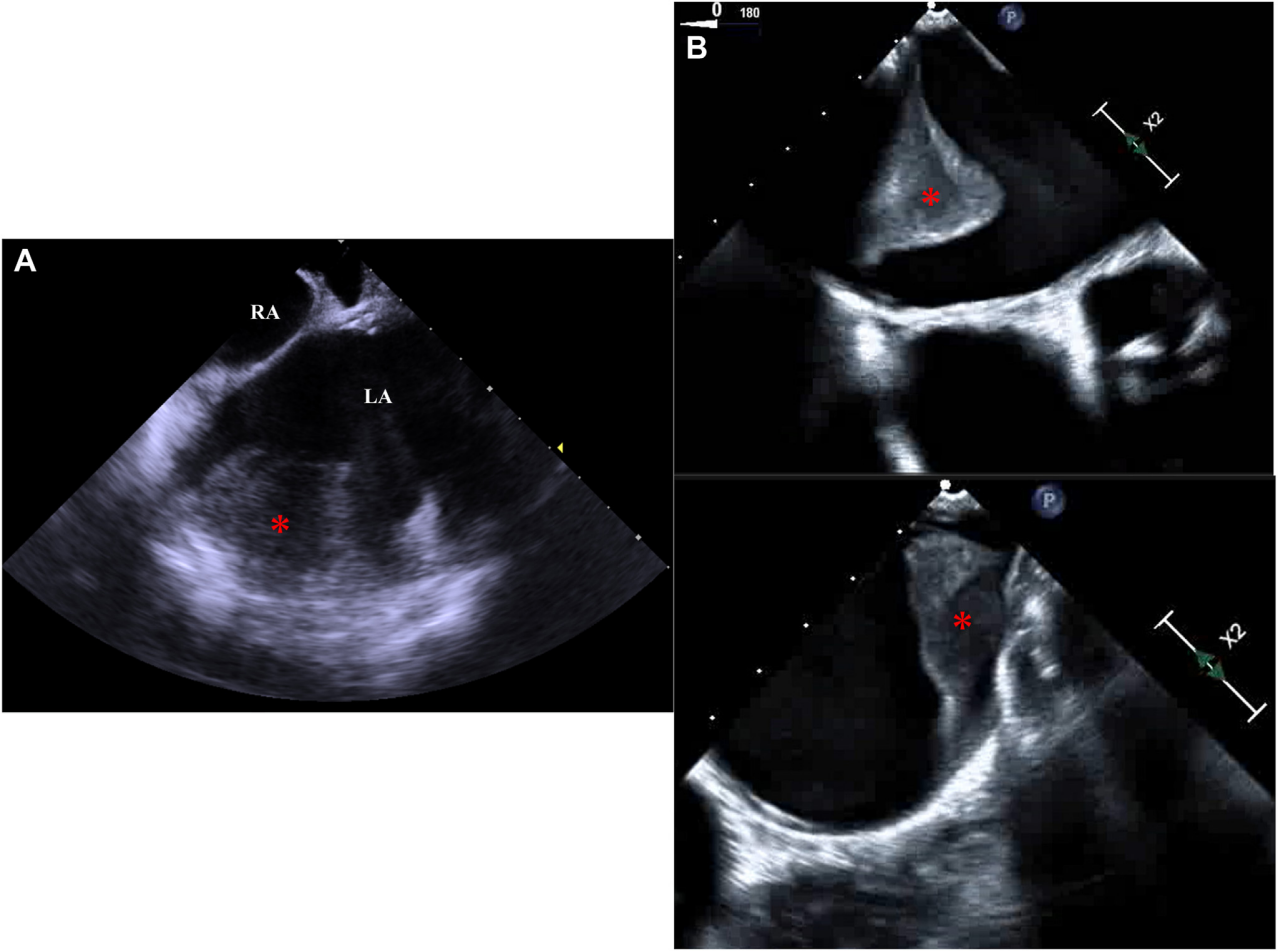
A: Intracardiac echocardiography revealed large thrombi (*asterix*) in the LA before the atrial septal puncture. B: Transesophageal echocardiography was performed to evaluate the LA thrombi; the 2 thrombi were 40.5 mm × 23.9 mm and 26.7 mm × 15.8 mm, respectively. RA = right atrium; LA = left atrium.
